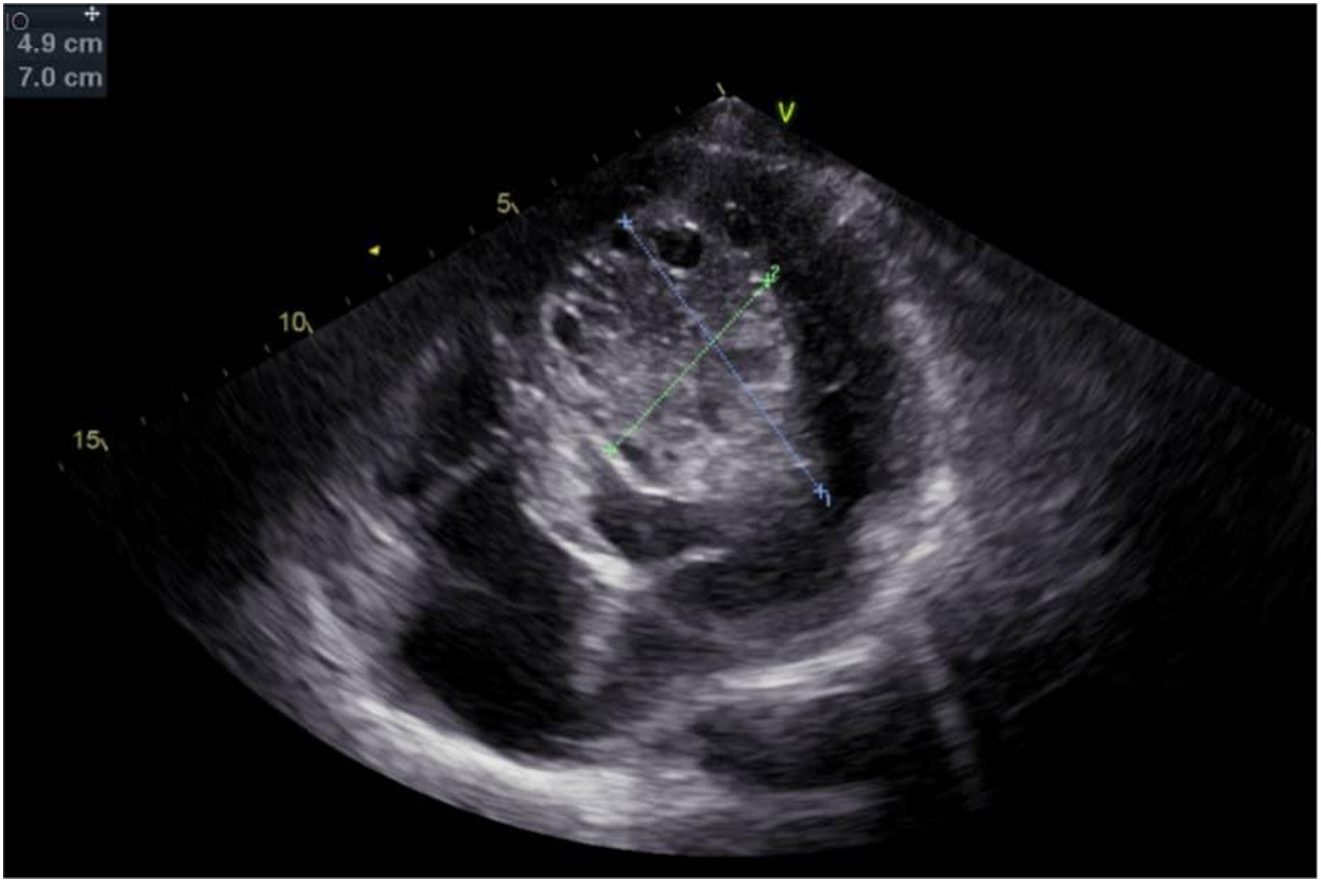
Four chamber TTE of a 35‐year‐old male with multi‐organ hydatid cyst. Severe LV enlargement with a septated echo‐free space (6.1 × 4.3 cm) in the LV cavity with mobile particles on its surface. LV, left ventricle; TTE, transthoracic echocardiography.
